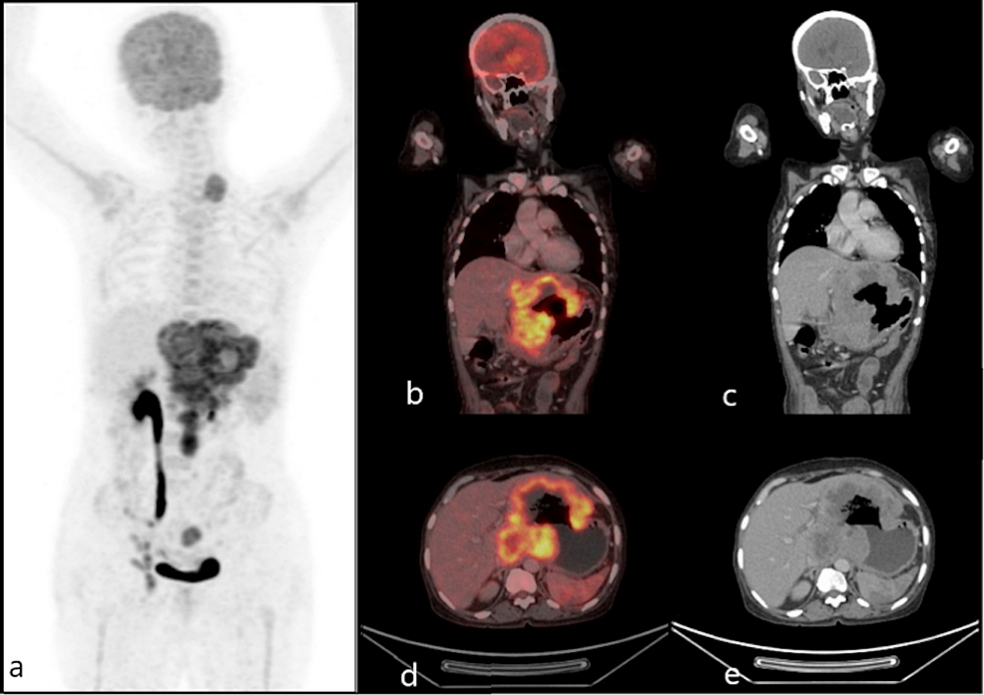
FDG PET-CT images showing (a) abnormally increased tracer uptake in the left supraclavicular region, stomach, liver, retroperitoneal region, and pelvis. Fused images (b-e) showing metabolically active lesion in segments II, III, and caudate lobes of the liver infiltrating the lesser curvature of the stomach resulting in a fistula. FDG, Fluoro-deoxy-D-glucose; PET, positron emission tomography; CT, computed tomography.
